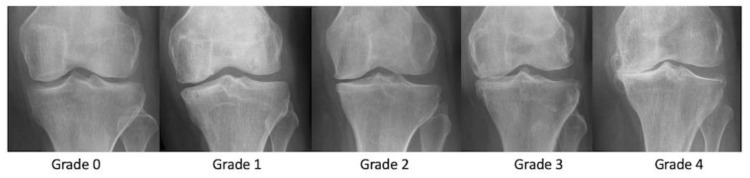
Anteroposterior radiograph of the left knee showing Kellgren–Lawrence grade 0, 1, 2, 3, and 4 OA.
